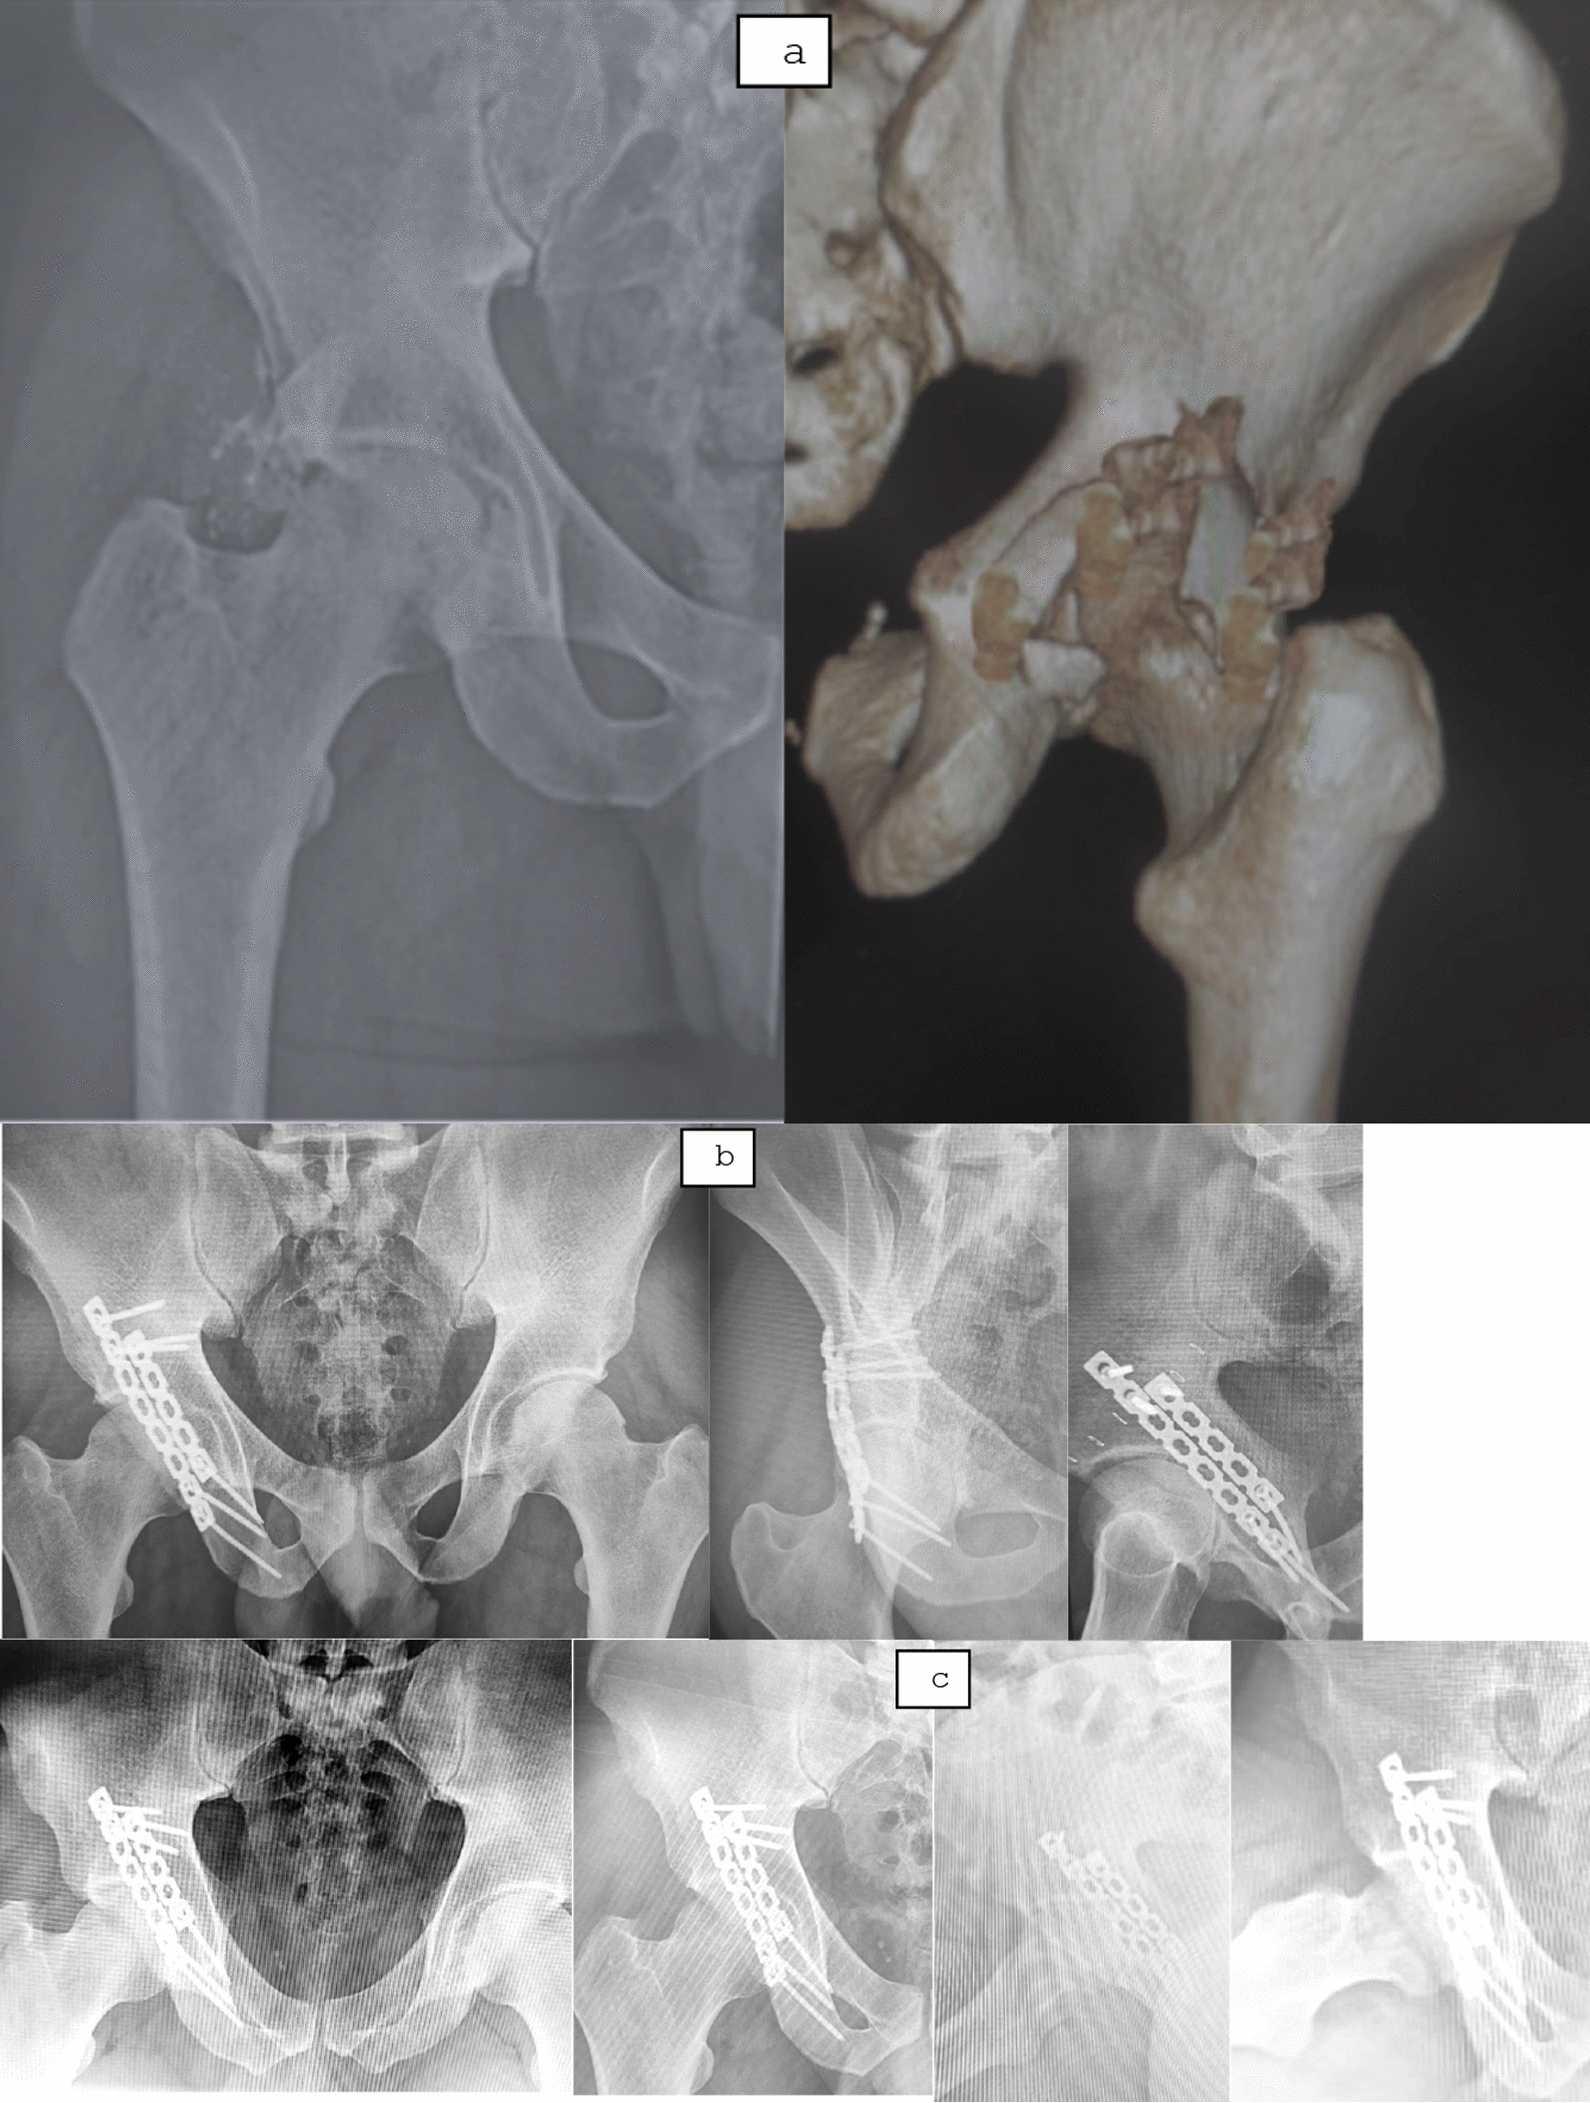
A 24-year-old male with a 6-week-old right comminuted posterior wall fracture. a Preoperative radiograph and 3D CT showed comminuted fracture with early callus formation. b Postoperative radiograph showing anatomical reduction with stable fixation. c Final 4 -year follow up radiograph showing maintained reduction with congruent hip
